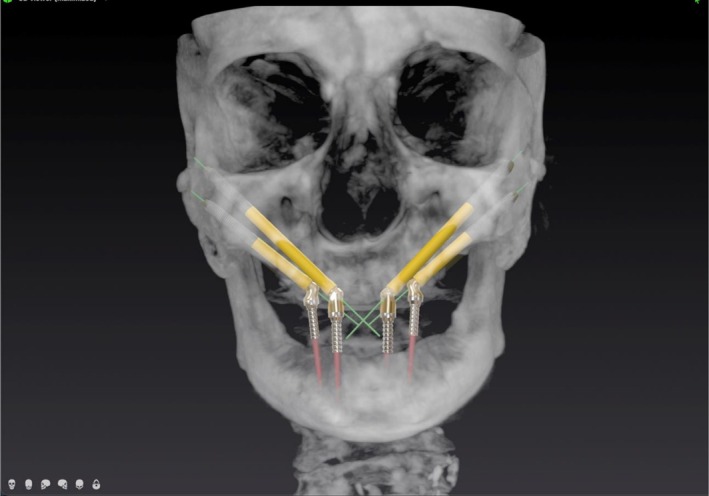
3D virtual surgical planning of quad zygoma implants based on CBCT data, defining implant trajectories according to anatomical constraints and optimal zygomatic apical anchorage.
